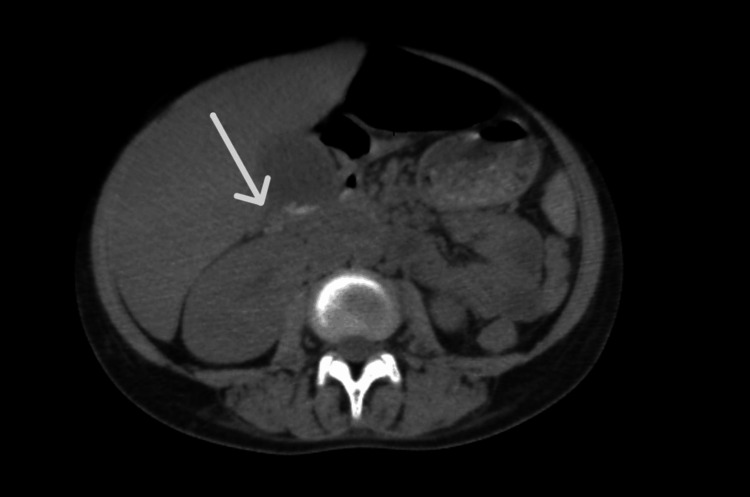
Contrast-enhanced abdominal CT scan demonstrating intrahepatic bile duct dilatation. Contrast-enhanced abdominal CT scan (axial view) showing marked dilatation of intrahepatic bile ducts (white arrow), consistent with cholestasis.
